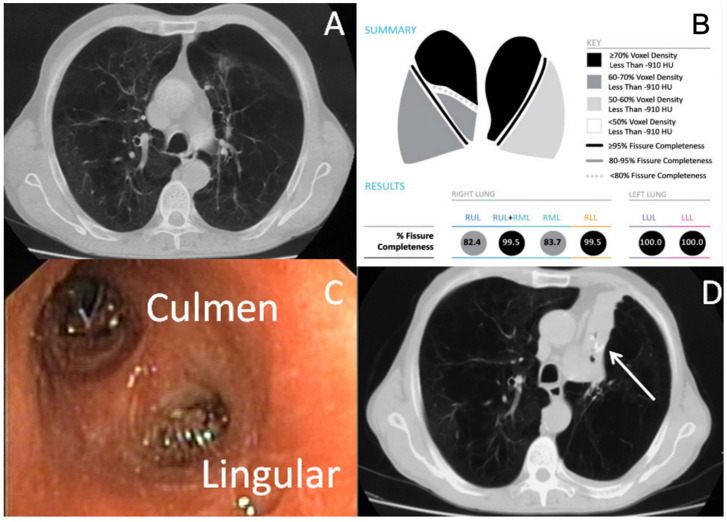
BLVR in a patient with heterogenous emphysema of LUL. (A): Computed Tomography scan shows heterogenous emphysema of upper lobes; (B): Quantitative computed tomography analysis shows complete fissure integrity between left upper lobe and left lower lobe; (C): Two valves were inserted to occlude the culmen and the lingular bronchus of the left upper lobe; (D): Complete collapse of left upper lobe was obtained after valve insertion (white arrow: endobronchial valve and collapsed left upper lobe).
